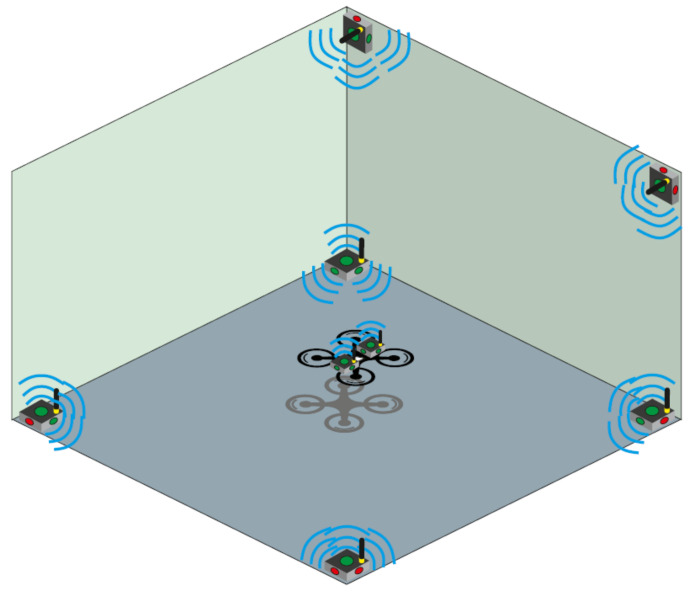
Concept of the ultrasound mobile beacon placement.
